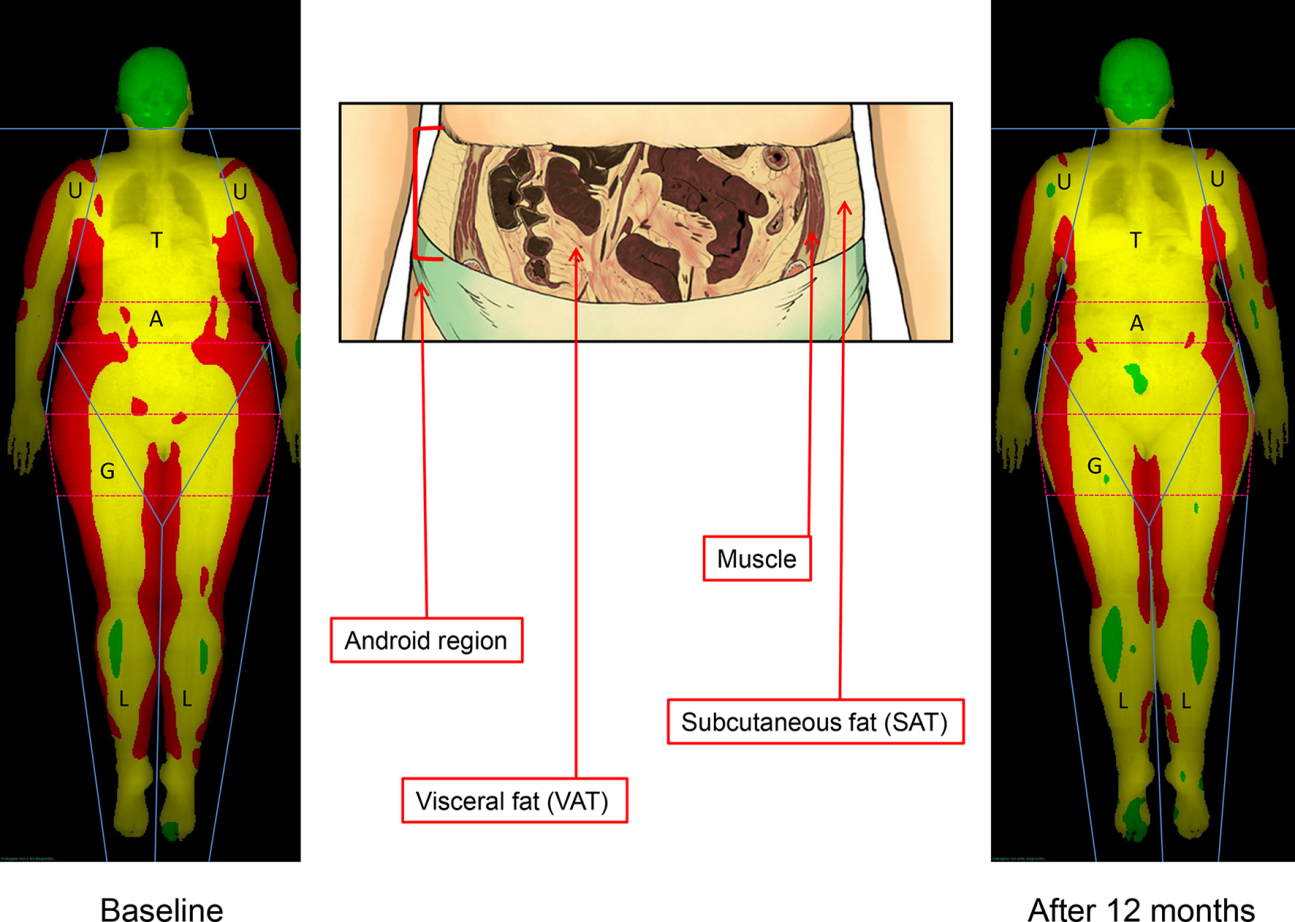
DXA body composition analysis. Colored soft tissues map of whole-body scan by DXA (red, high fat percentage—conventionally >60%; yellow, medium fat percentage—between 25 and 60%; green, low fat percentage—<25%) of the subject A3, at baseline (left) and after 12 months (right), in order to outline regional changes of body composition (trunk,T; upper limbs, U; lower limbs, L; android, A [the portion of the abdomen included between the line joining the two superior iliac crests and extending cranially up to the 20% of the distance between this line and the chin]; gynoid, G [the portion of legs stretching caudally from the femoral great trochanter to a distance double of the android region]). An enlargement of the android region is also presented (center) with the representation of the abdominal muscles and subcutaneous and visceral fat compartments (anatomic picture by Andrea Plazzi, Imola, Italy). DXA, dual-energy X-ray absorptiometry; SAT, subcutaneous adiposetissue; VAT, visceral adipose tissue.
